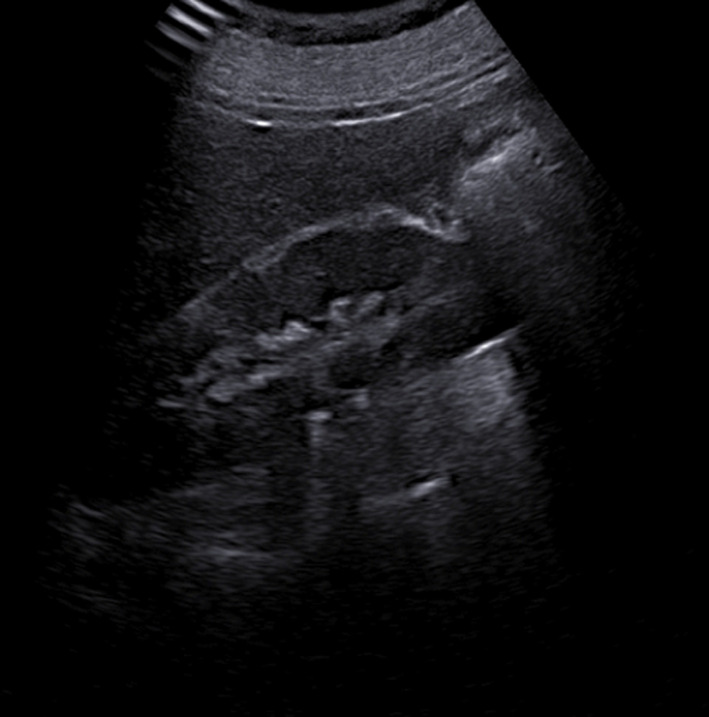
postoperative ultrasound imaging of the left kidney
